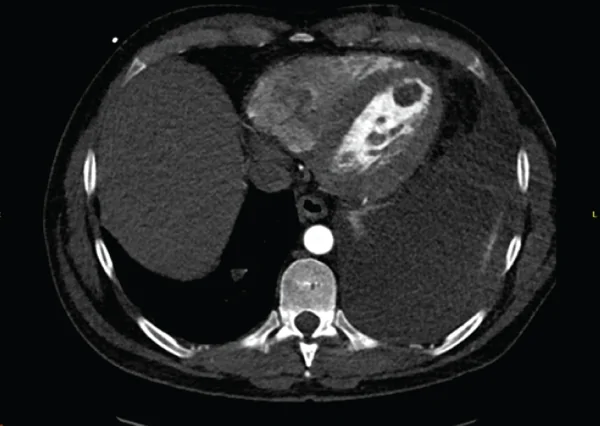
Axial slice of CT‐coronary angiogram on Day 9 of admission.
